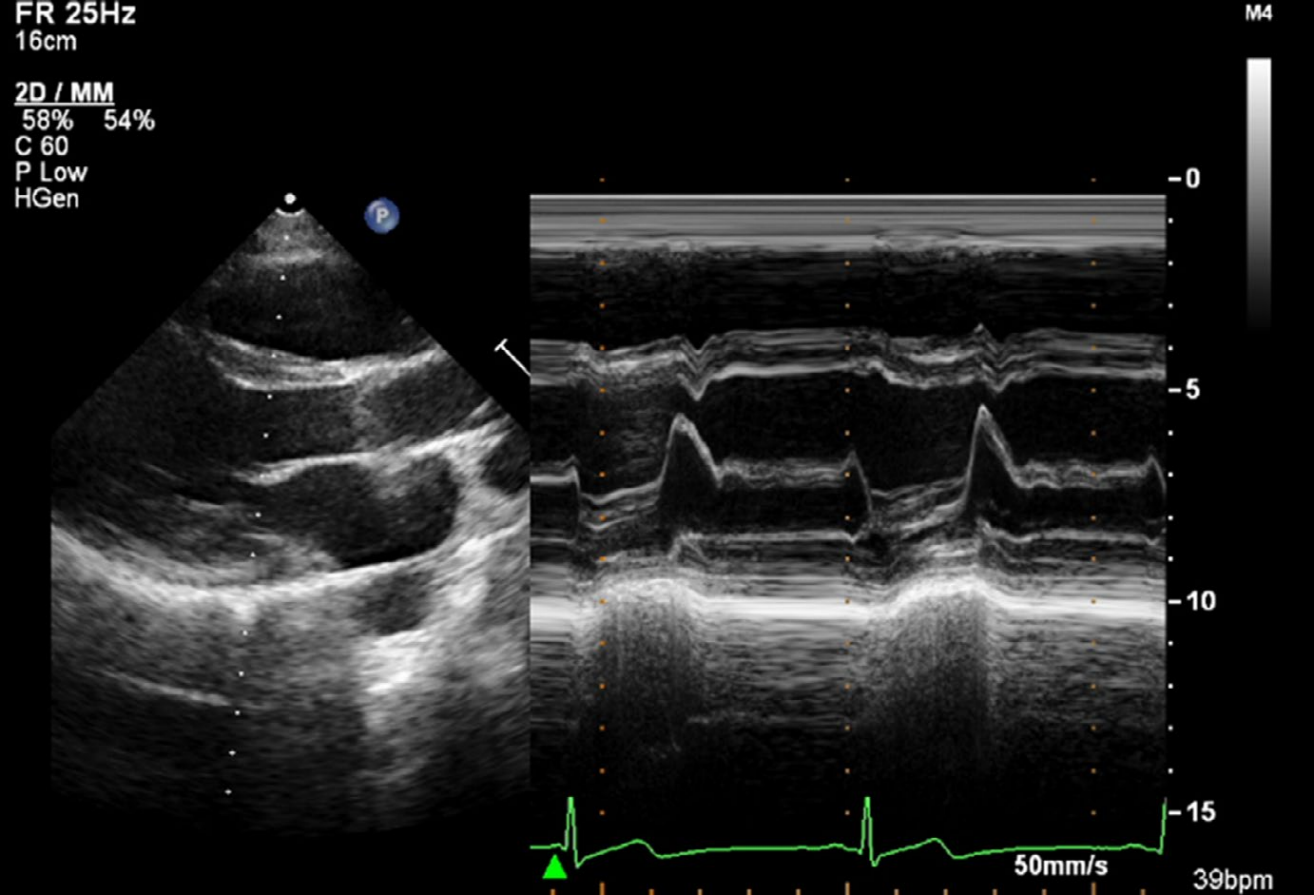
Shows echocardiography. No structural heart disease is observed
